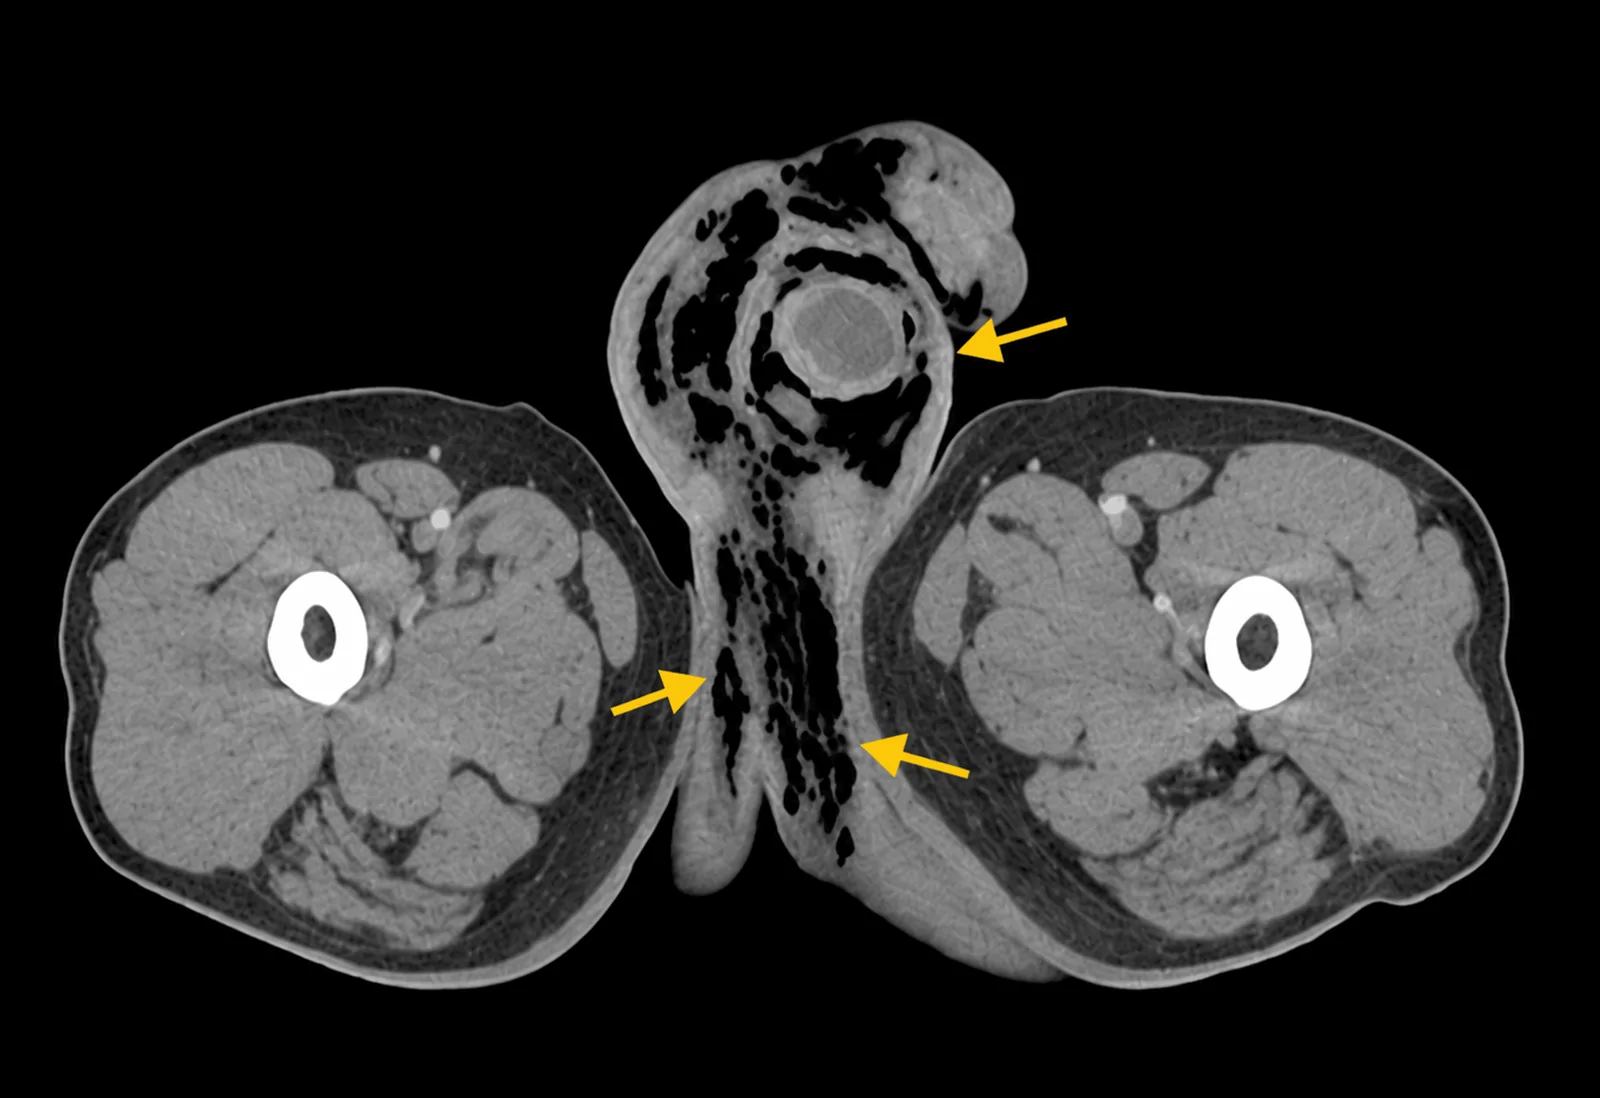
CT findings at presentation Axial contrast-enhanced CT image demonstrating extensive subcutaneous emphysema involving the scrotal and perineal soft tissues with extension along fascial planes (arrows), consistent with necrotizing soft tissue infection in Fournier's gangrene. CT: computed tomography
